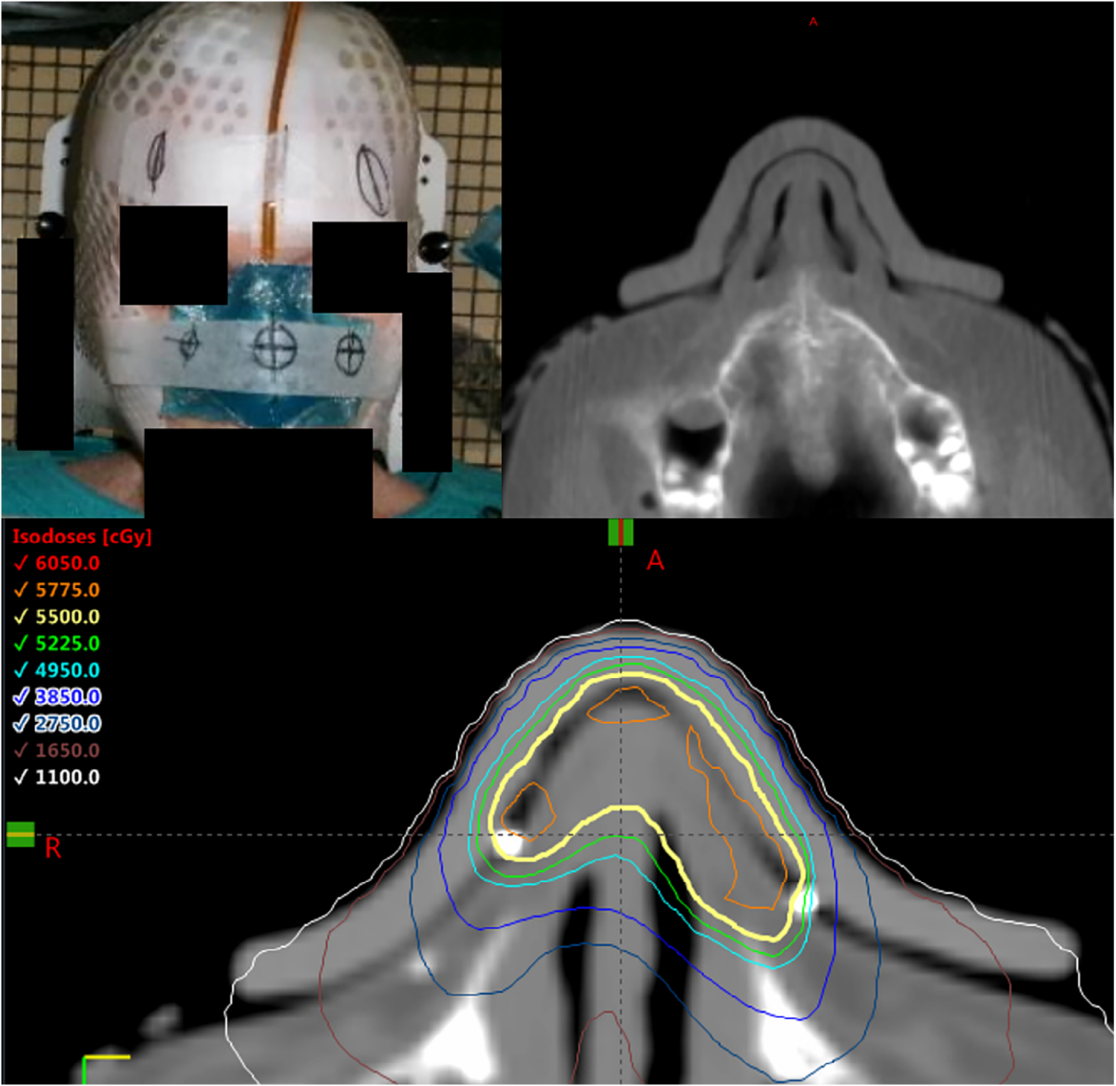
Photos of the clinical case study. Top left image shows the 3D printed bolus fitted on the patient. Top right image shows the planning CT of the patient with the bolus fitted. Bottom image shows the isodose distribution for the VMAT plan.
